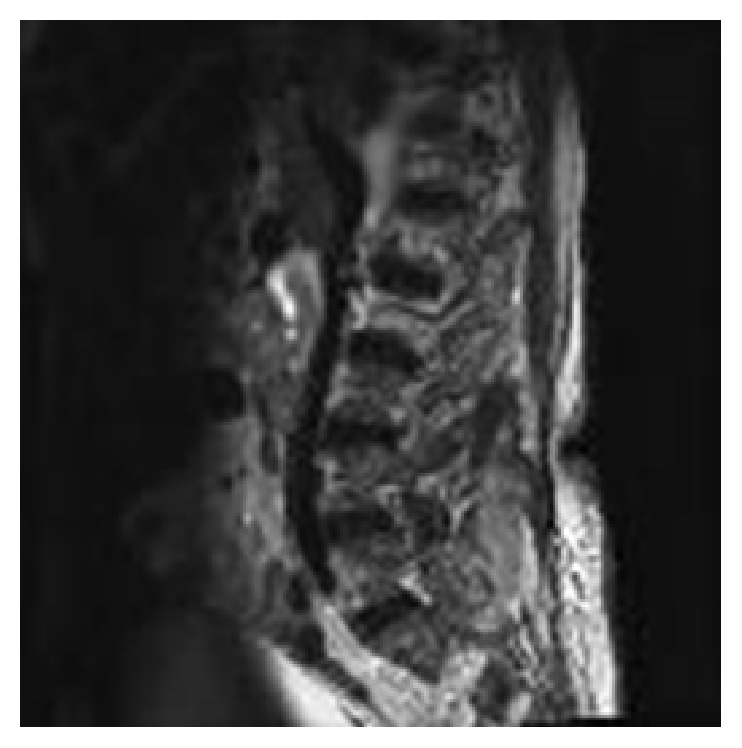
MRI: sagittal thoracolumbar spine T2.
